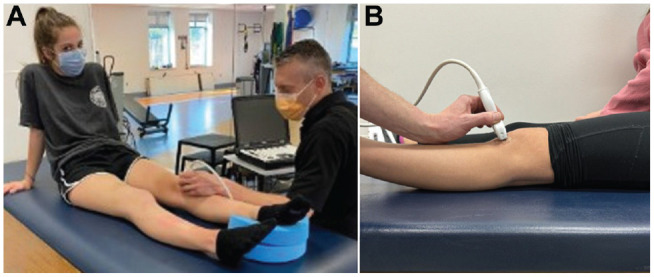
(A) Patient positioning for US measurement. (B) Lateral view showing probe orientation. US, ultrasound.
